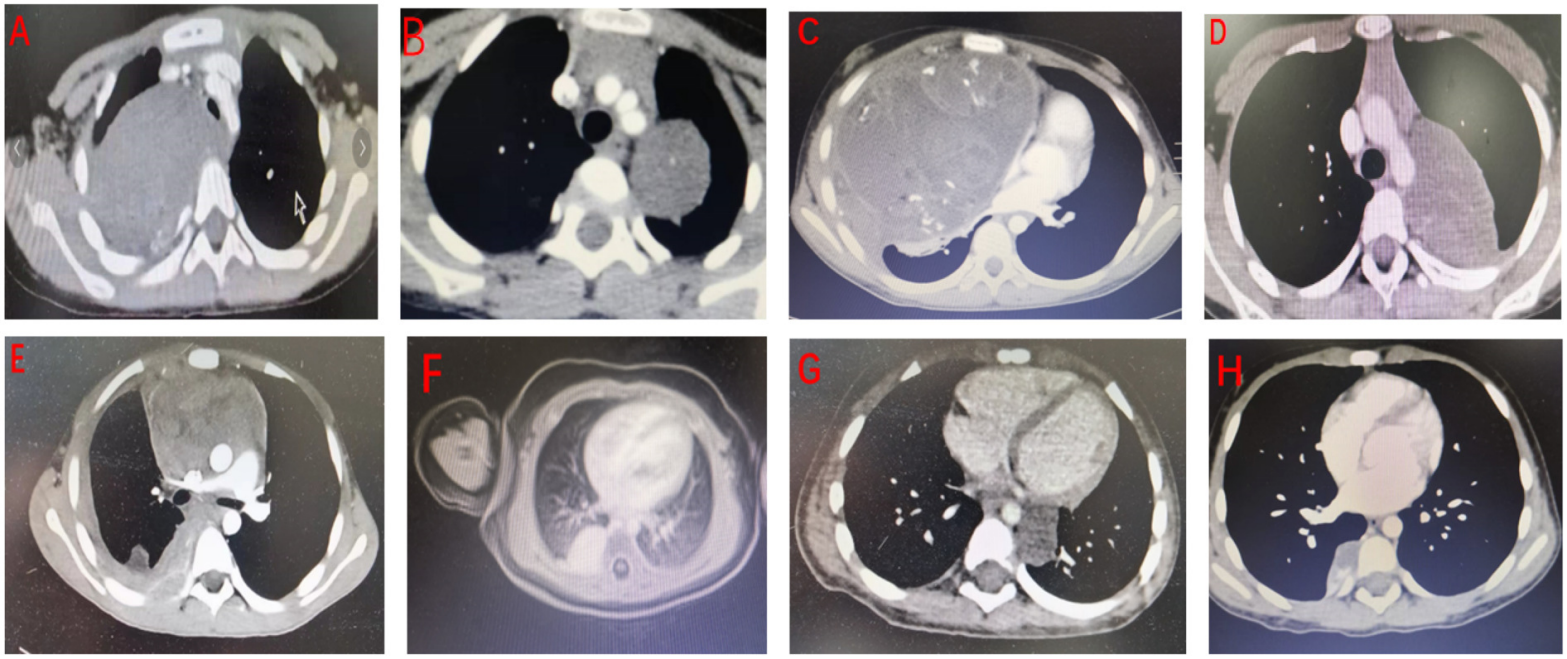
Selected CT images of mediastinal masses from representative patients. Postoperative pathological diagnoses are as follows: (A) ganglioneuroma; (B) ganglioneuroma; (C) mixed germ cell tumor (yolk sac tumor and mature teratoma); (D) schwannoma; (E) lymphoma; (F) hemangioma; (G) esophageal duplication cyst; (H) extralobar pulmonary sequestration.
